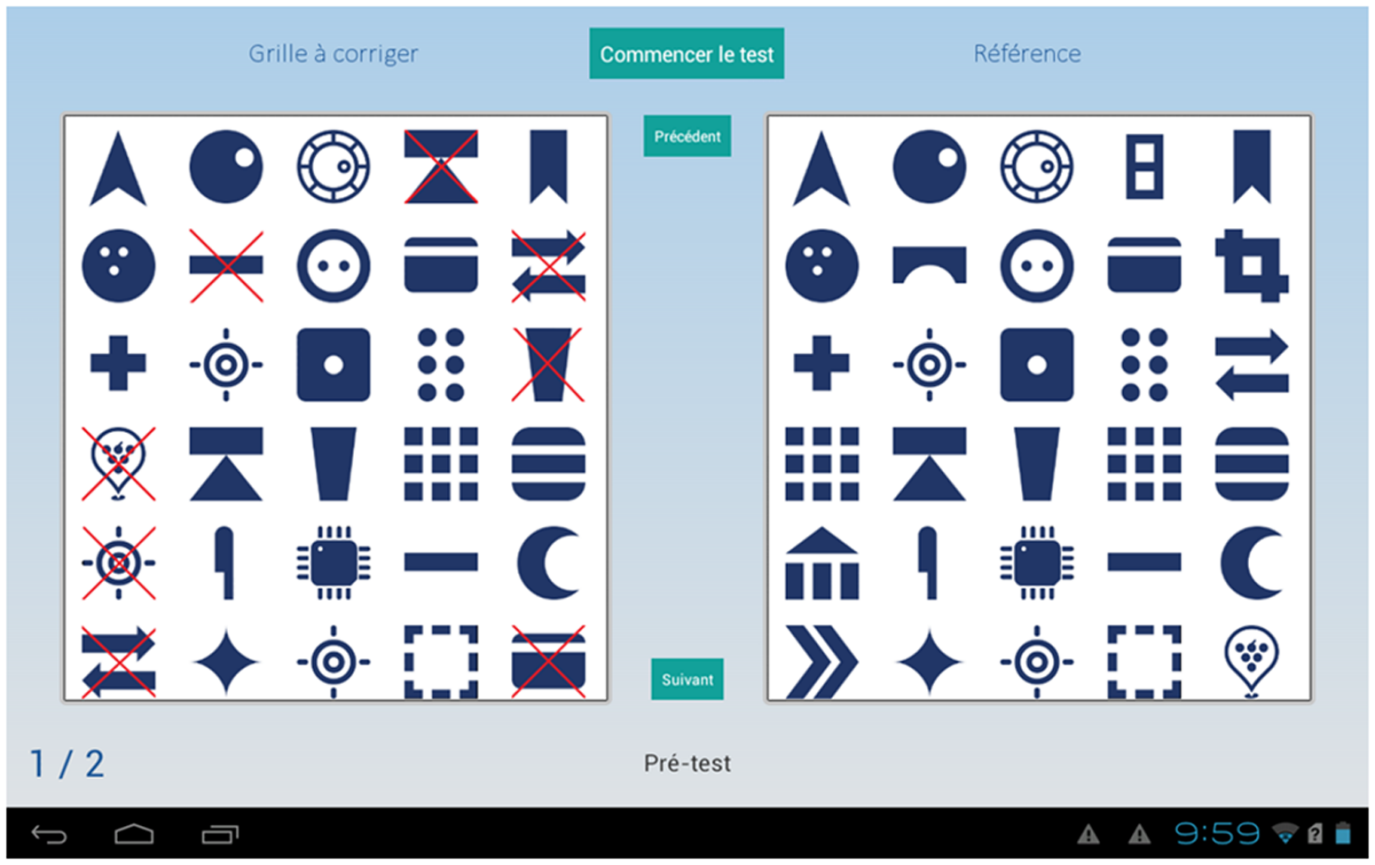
Table-based cancellation test (e-CT).
